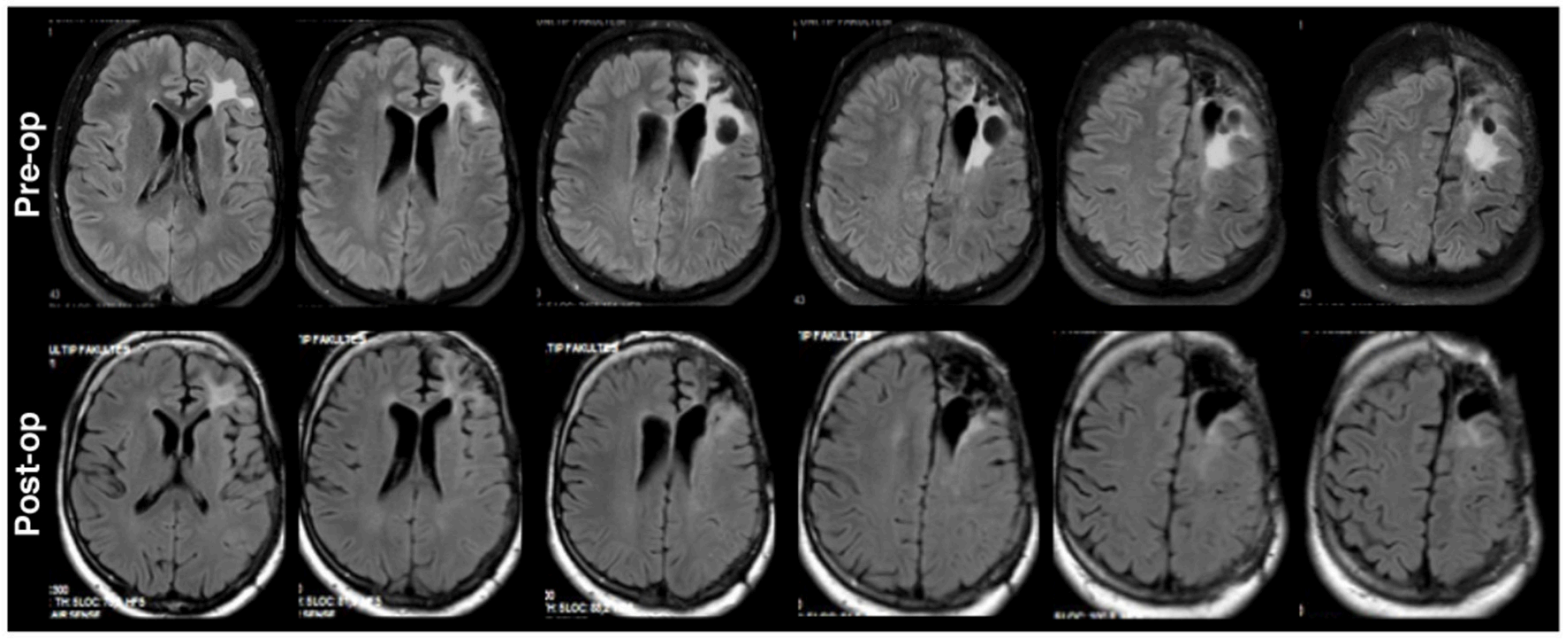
Axial fluid-attenuated inversion recovery (FLAIR) brain MRI for P1 shown in radiological convention. Preoperative left frontal hyperdensities (upper) around the previous operation tumor space were reduced in size in postoperative MRI (lower).
